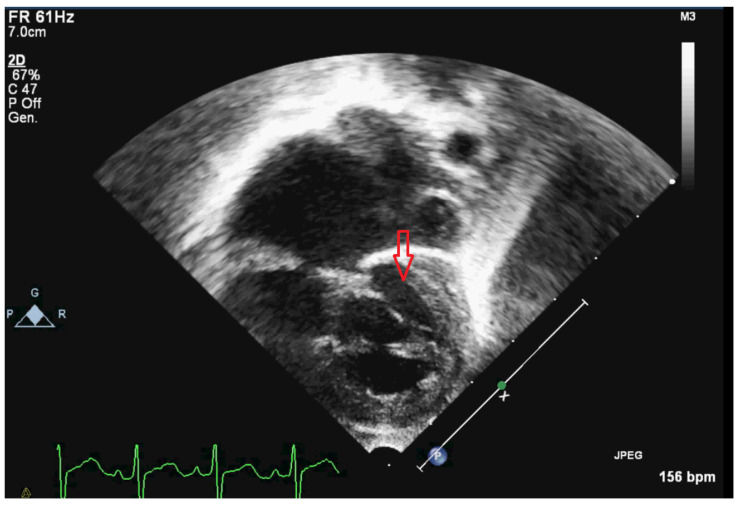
Apical four chambers view showing the left “slit like” ventricle and the atresia of the mitral valve plane. The arrow points at the left ventricle.
